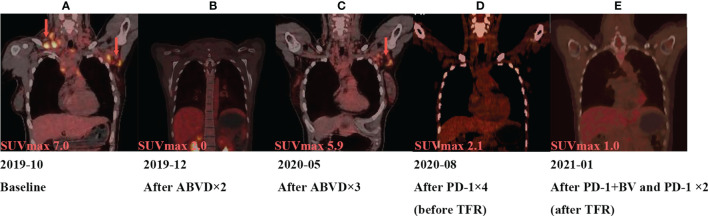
Tumor assessment during treatment by PET. (A) In October 2019, the size of the left axillary lymph node was 3.1 × 2.2 cm, and maximum standard uptake value (SUVmax) from 18F-FDG is 7.0. (B) In December 2019, the size of the left axillary lymph node was 1.51 × 0.84 cm, SUVmax is 2.0. (C) In May 2020, the left axillary lymph node was1.69 × 1.69 cm, and SUVmax is 5.9. (D) In August 2020, the left axillary lymph node was 1.5 cm, and SUVmax is 2.1. (E) In January 2021, PET/CT showed the SUVmax is 2.1.
